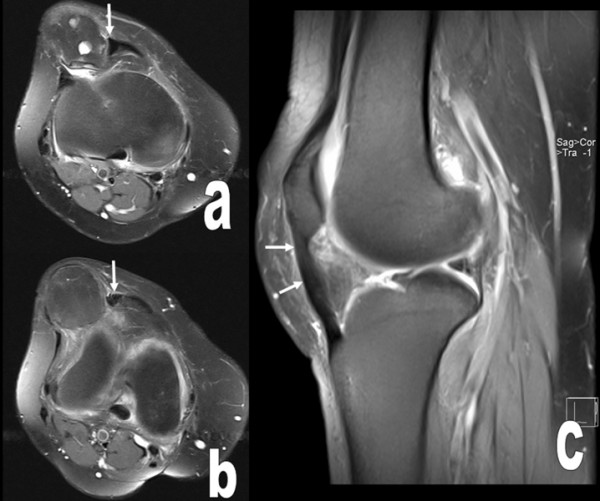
The osteochondral mass within the retinaculum, its compression to the patellar tendon, increased thickness and signal of the patellar tendon (arrows) are seen at axial fat saturated proton density (a, b) and sagittal fat saturated T2 weighted MR images (arrows) (c).
